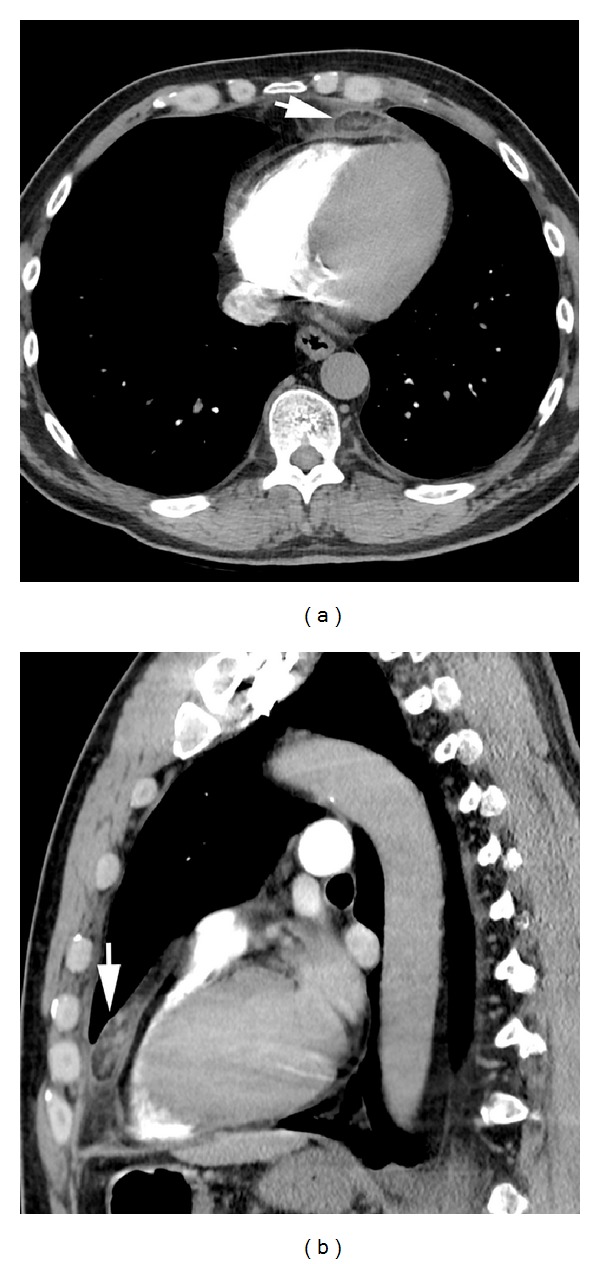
Axial (a) and sagittal (b) contrast-enhanced chest CT (soft tissue window) shows an anterior mediastinal ovoid fat attenuation lesion (arrows) with intrinsic and surrounding soft tissue attenuation stranding. The lesion is demarcated by a thin rim of soft tissue attenuation and abuts the adjacent pericardium. Note small left pleural effusion (b).
